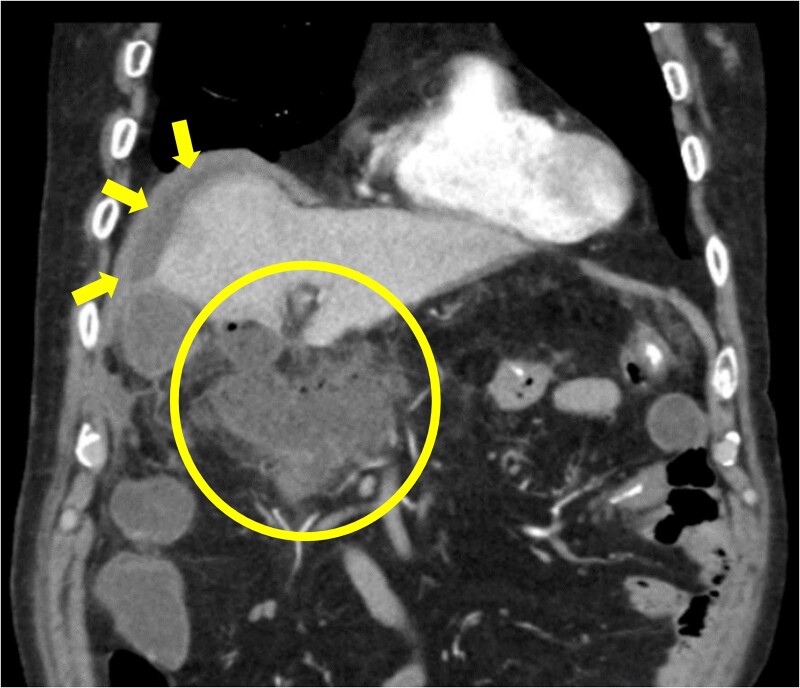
Diagnosis of postoperative duodenal perforation. CT showed ascites in subdiaphragmatic space (arrow) and around duodenal stump (circle) at POD 8.
